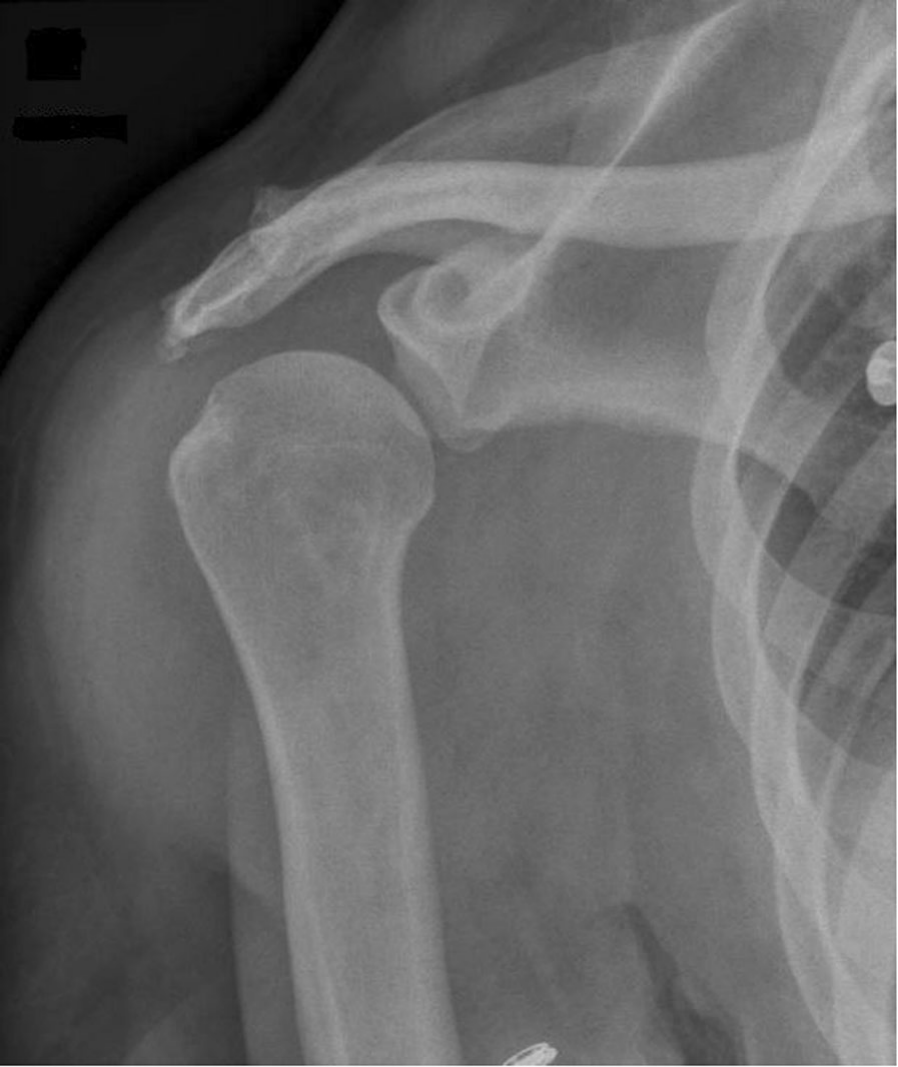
Pre-operative AP right shoulder Xray without degenerative signs
